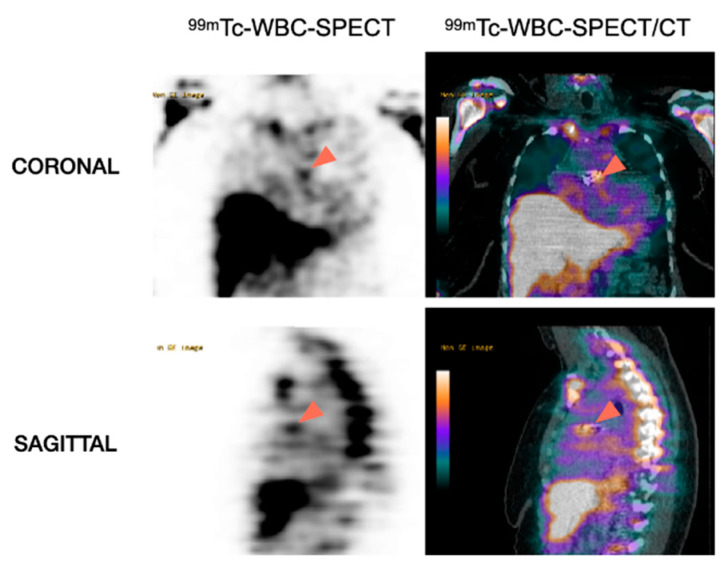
Role of WBC-SPECT/CT for the detection of prosthetic valve endocarditis. Note the accumulation of ^99m^Tc-labeled WBC in the region corresponding to the aortic prosthetic valve (arrowheads) in favor of PVE.
